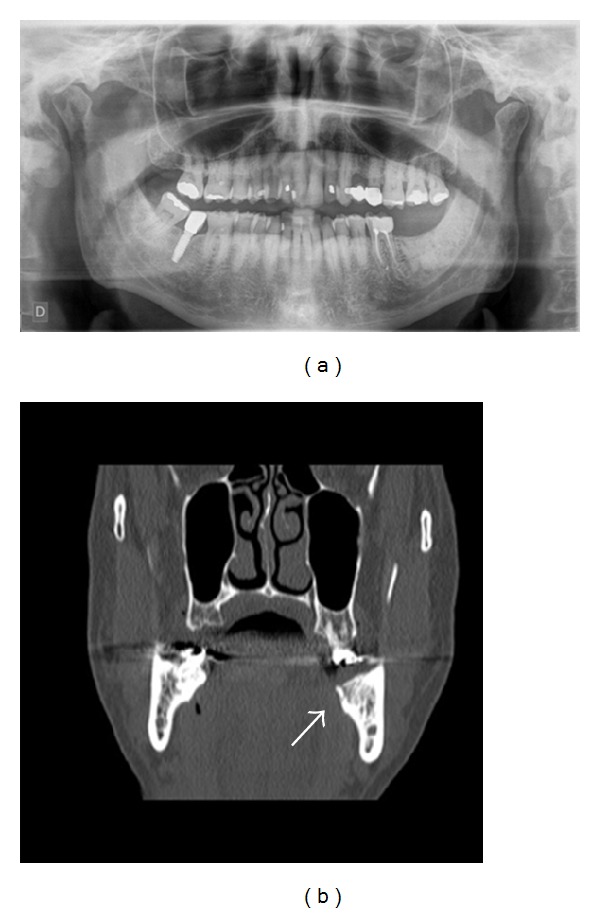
(a) Panoramic radiograph. Notice in the affected area a high bone density image. (b) CT image. Notice a lingual thin fissure line in the affected area.
